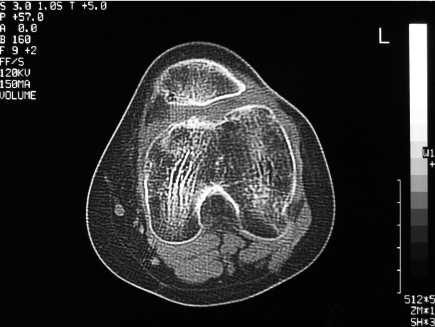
Postoperative computed tomography demonstrating no malalignment of the patella and improvement of sulcus angle from 170° to 140°.
